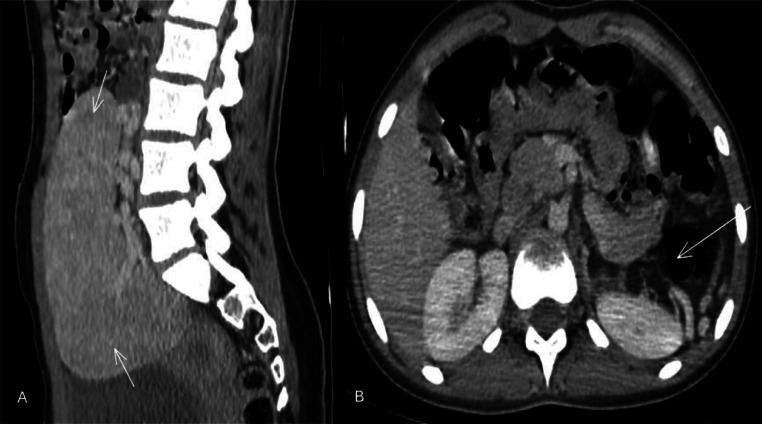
Contrast-enhanced axial and sagittal CT images of the upper abdomen showing the absence of splenic tissue in the left upper quadrant, with an enlarged ectopic spleen located in the pelvic region and a mild delay in homogenization during the portal phase.
